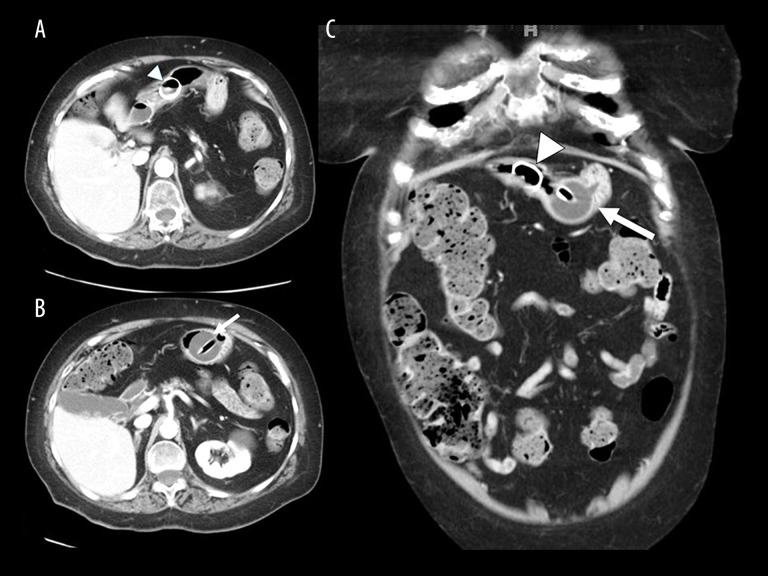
Axial and coronal CT images of the abdomen and pelvis demonstrate a round, radiodense disc in the gastric lumen (arrowhead in A and C), not connected to the balloon-retained PEG tube (long arrow in B and C).
